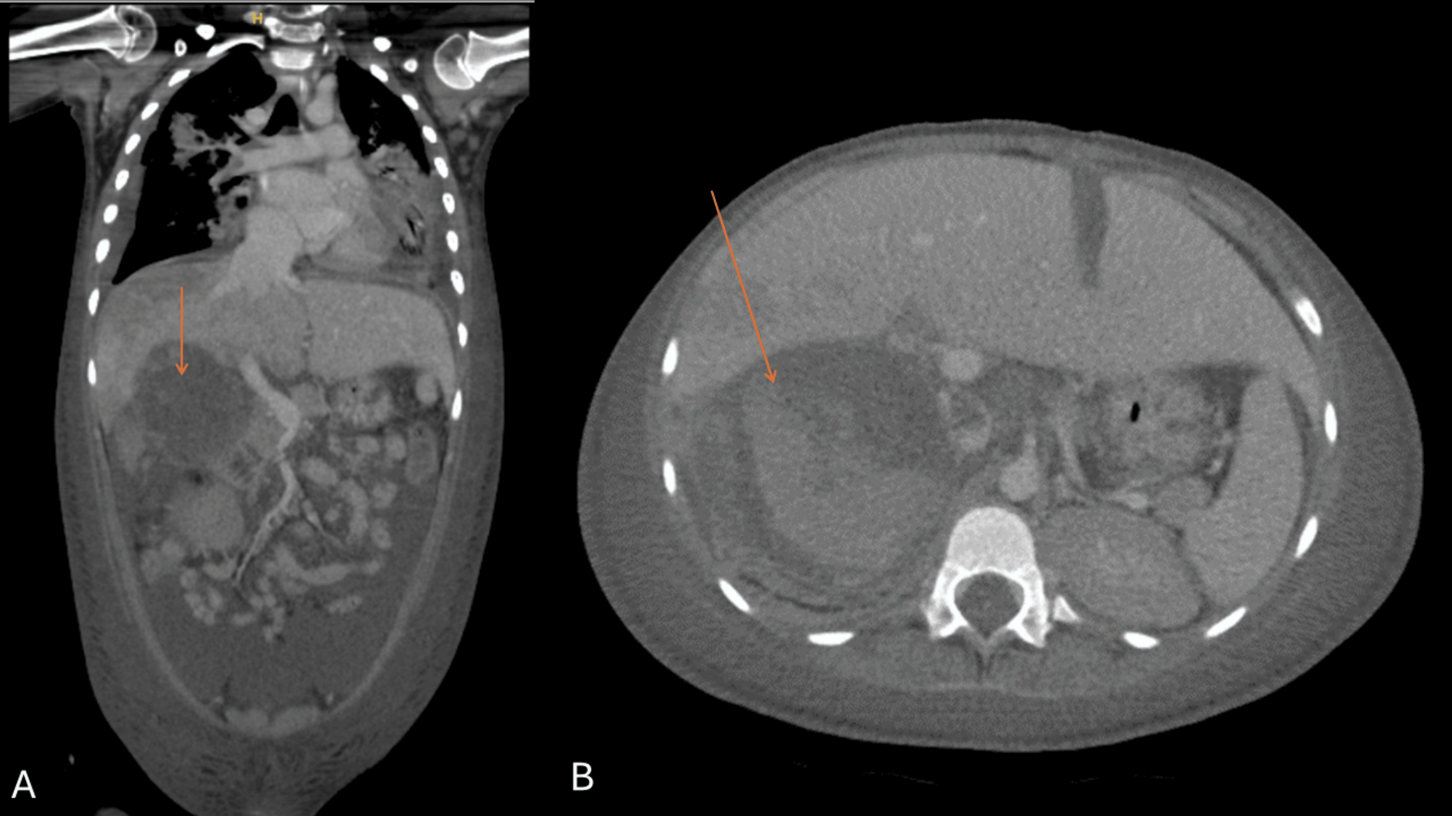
Contrast-enhanced full body CT scan showing the right adrenal mass A: coronal and B: axial view of the CT scan. Both show a right sided adrenal tumor, indicated by the orange arrow, demonstrating heterogenous enhancing and non-enhancing components.
